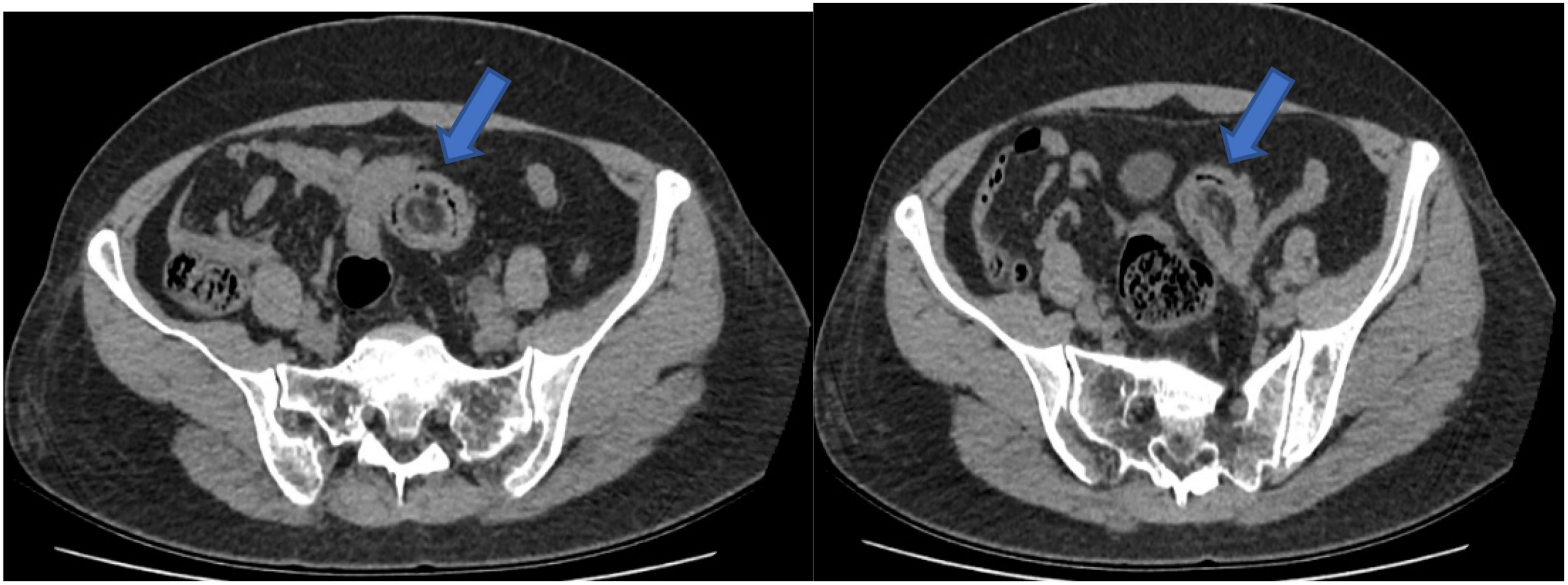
Axial slice of computed tomography showing intussusception (arrow).
